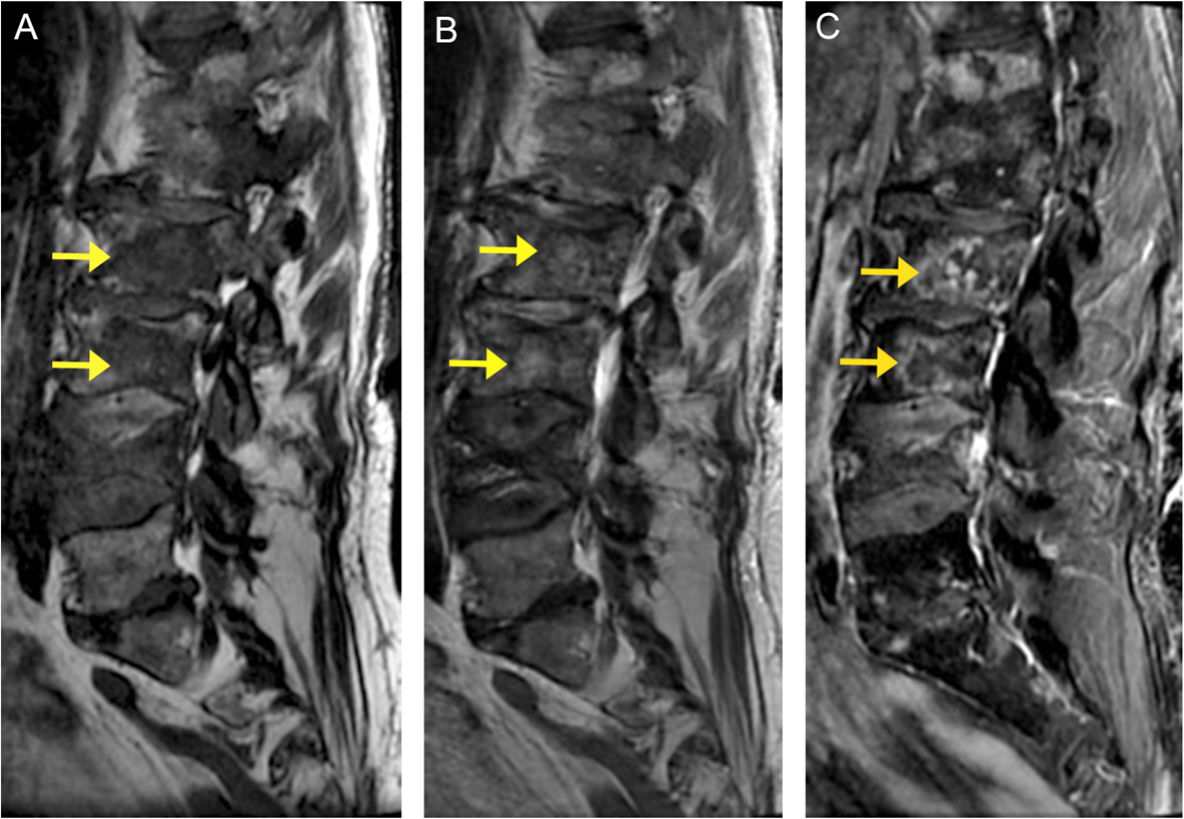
Spinal metastases. Sagittal MR images demonstrate numerous ill-defined lesions throughout the lumbar spine (arrows). These lesions demonstrate low signal on the T1-weighted sequence (a), intermediate to high signal on the T2 -weighted sequence (b), and heterogenous contrast enhancement on the post-contrast T1 fat-saturated sequence (c)
